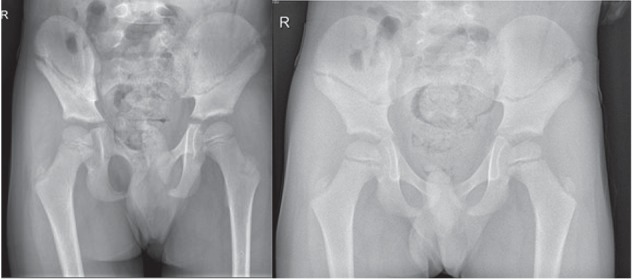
Radiography showed stable condition after three weeks.
